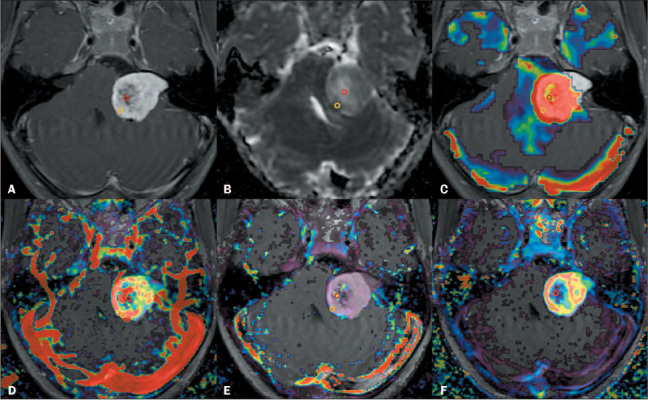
MRI scans of a 41-year- old female with a left-sided Hannover T4 cystic VS. Images
